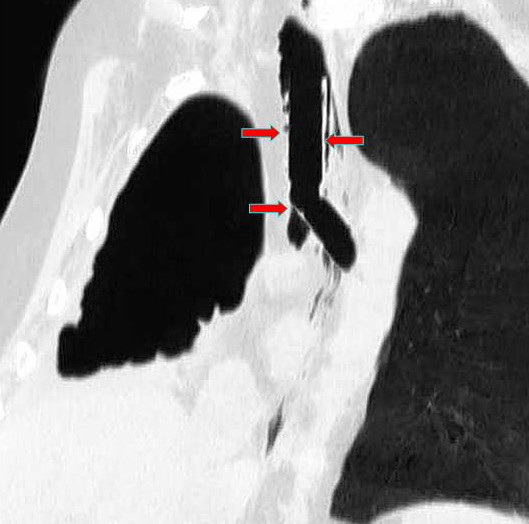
CT multiplanar reformation (MPR), oblique coronal view, after stent placement. The tracheal and bronchial arms of the Dumon stent (red arrows) tightly adhere to the tracheobronchial wall. The covered portion of the stent completely excludes the bronchial stump of about 1 cm length.
